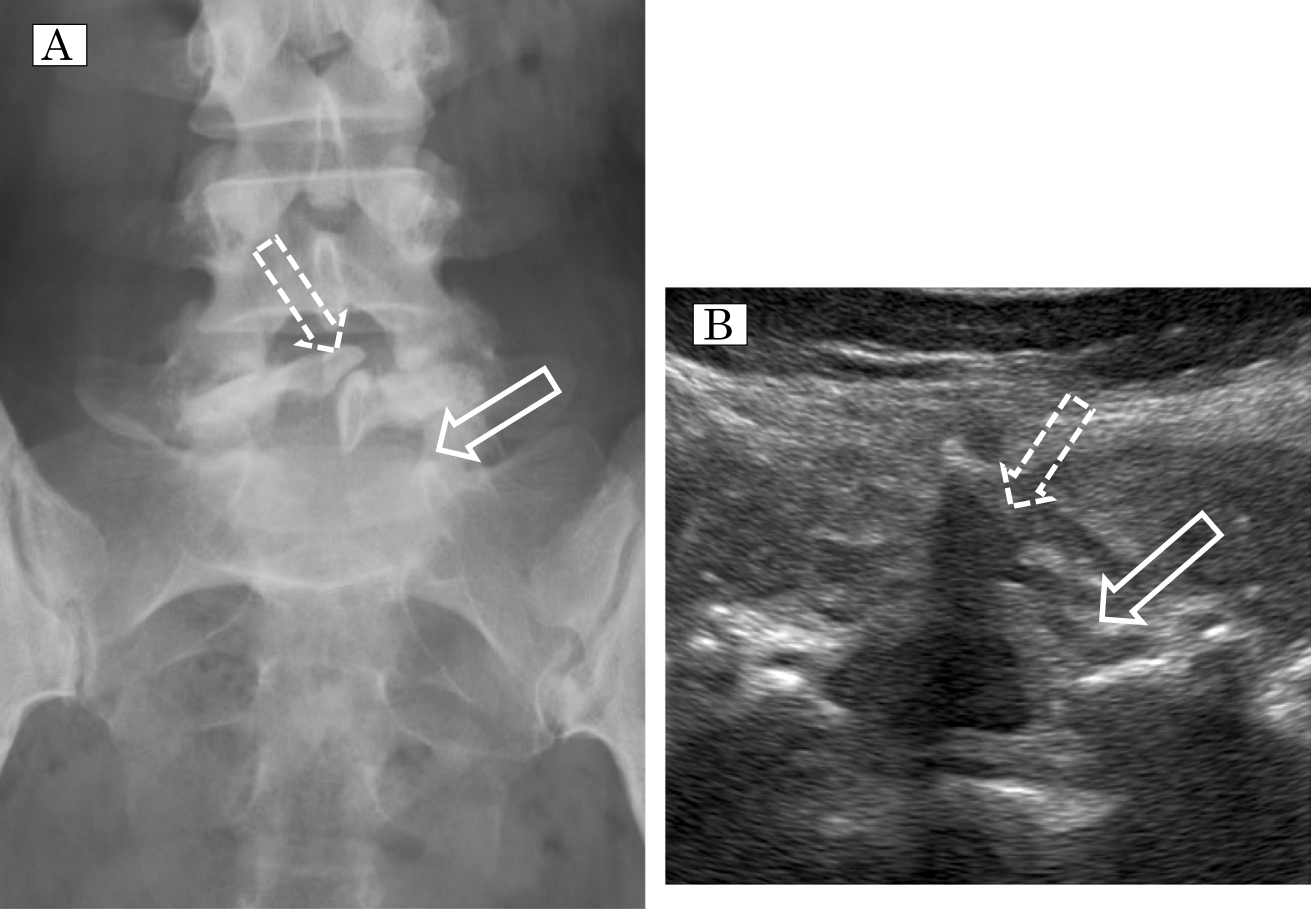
A Radiographical image of the lumbar spine on AP view. The solid arrow shows the partial defect of the vertebral arch of at the L5 level. The dashed arrow shows incomplete fusion of the spinous process at L5; higher lumbar levels are unaffected. B Ultrasonographic image of axial view at the level of L4/5. The solid arrow shows incomplete fusion of the vertebral arch at L5. The dashed arrow shows the incomplete fusion of the spinous process of the L5 vertebra
